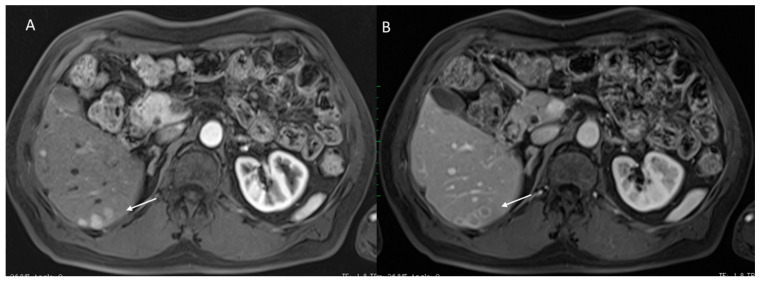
HCC on VI seg. MRI assessment. The lesion (arrow) shows APHE during arterial phase (A) with washout and capsule appearance (B) during portal phase of contrast study.
